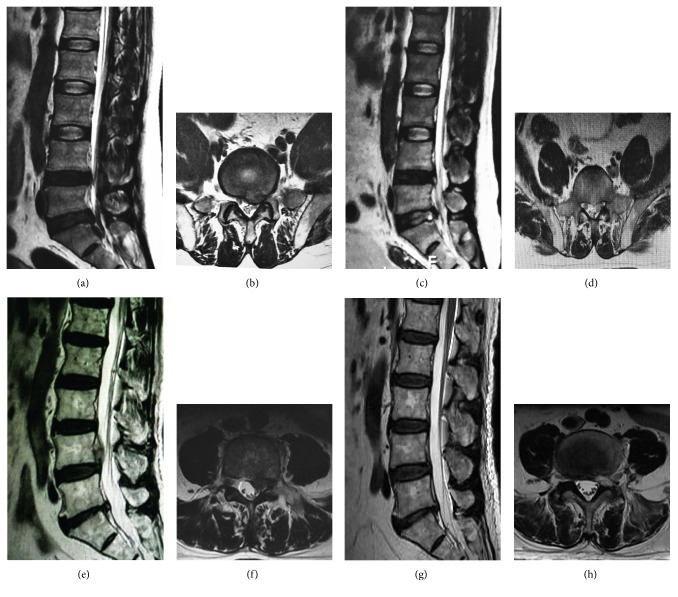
Preoperative and postoperative imaging examinations. (a, b) Preoperative MRI revealed L5/S1 disc herniations with nucleus pulposus highly downward migrated to S1 intervertebral space. (c, d) Postoperative MRI examination revealed clean removal of the nucleus pulposus. (e, f) Preoperative MRI revealed L4/5 disc herniations with nucleus pulposus highly upward migrated to L4 vertebral posterior. (g, h) Postoperative MRI examination revealed clean removal of the nucleus pulposus.
